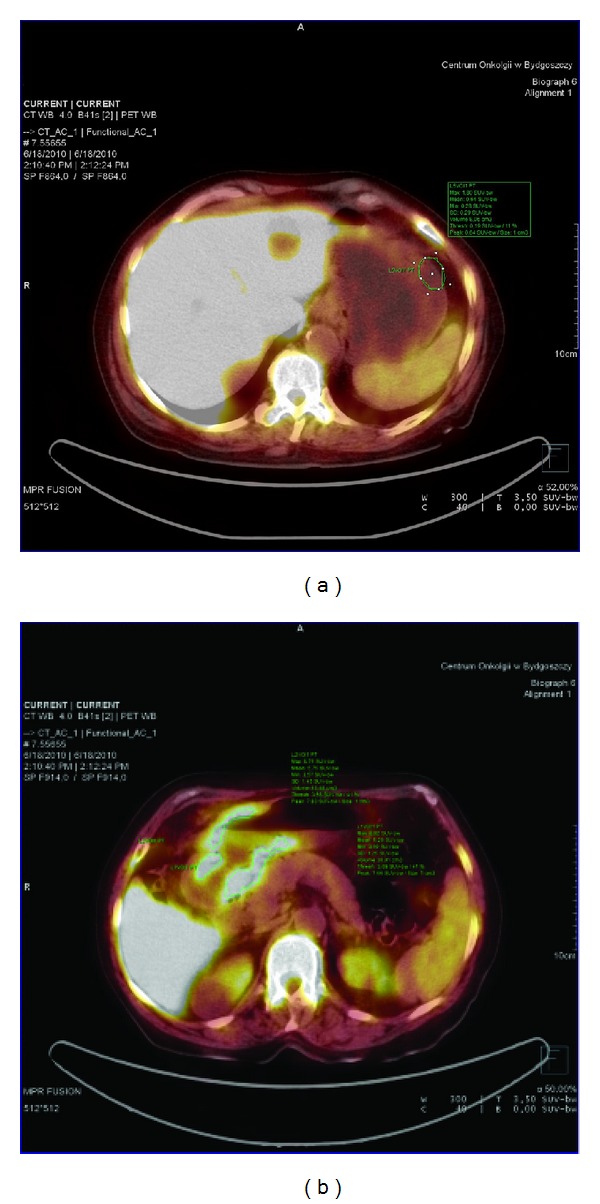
(a) ^18^FLT-PET in patient with undifferentiated adenocarcinoma. SUV_max⁡_ in normal gastric wall was 1.8. (b) ^18^FLT-PET in patient with undifferentiated adenocarcinoma. SUV_max⁡_ = 8.9.
